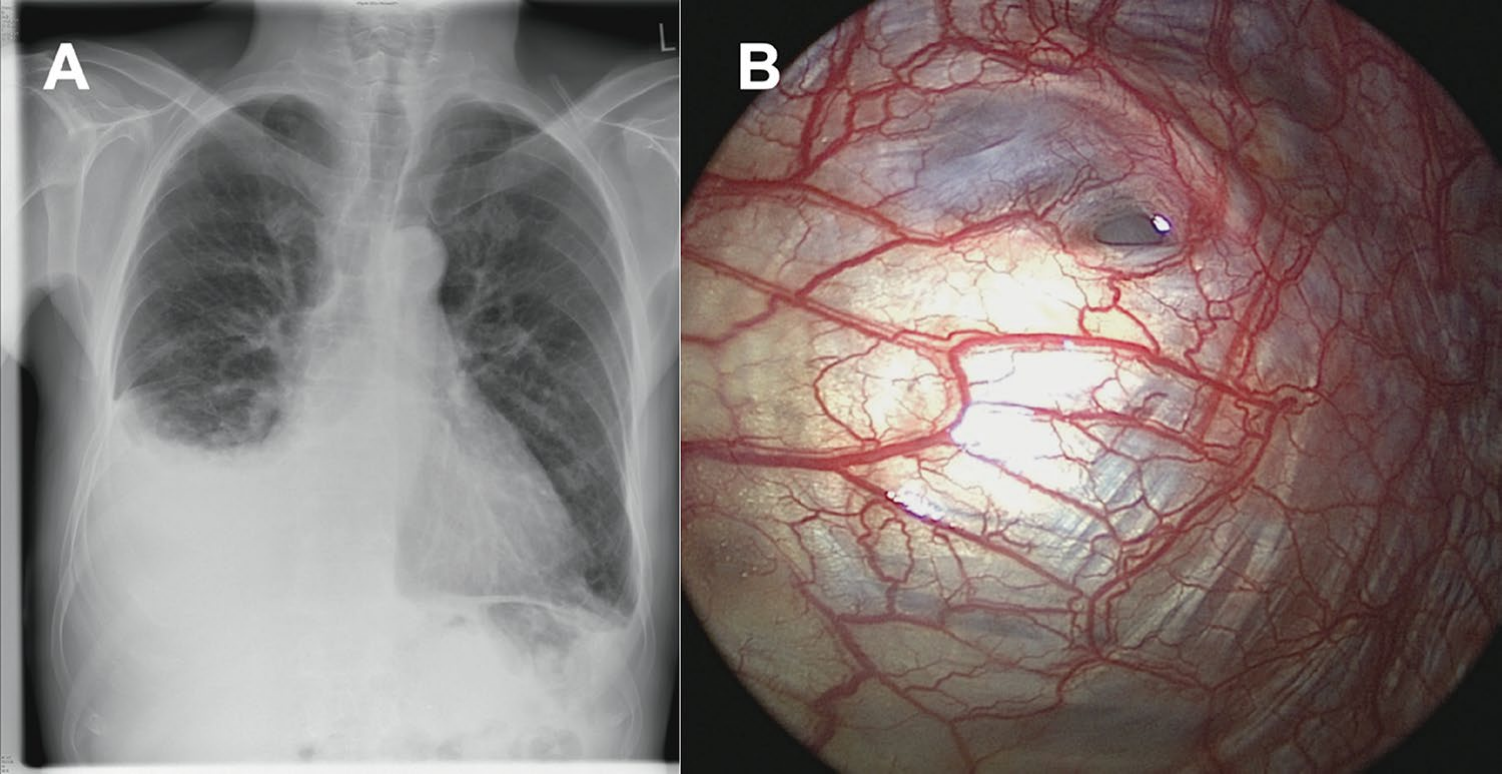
Right-sided pleuroperitoneal leakage in a 74-year-old male peritoneal dialysis patient. A Chest x-ray showing a right-sided pleural effusion. B Pleuroperitoneal leakage site demonstrated during video-assisted thoracoscopic surgery
